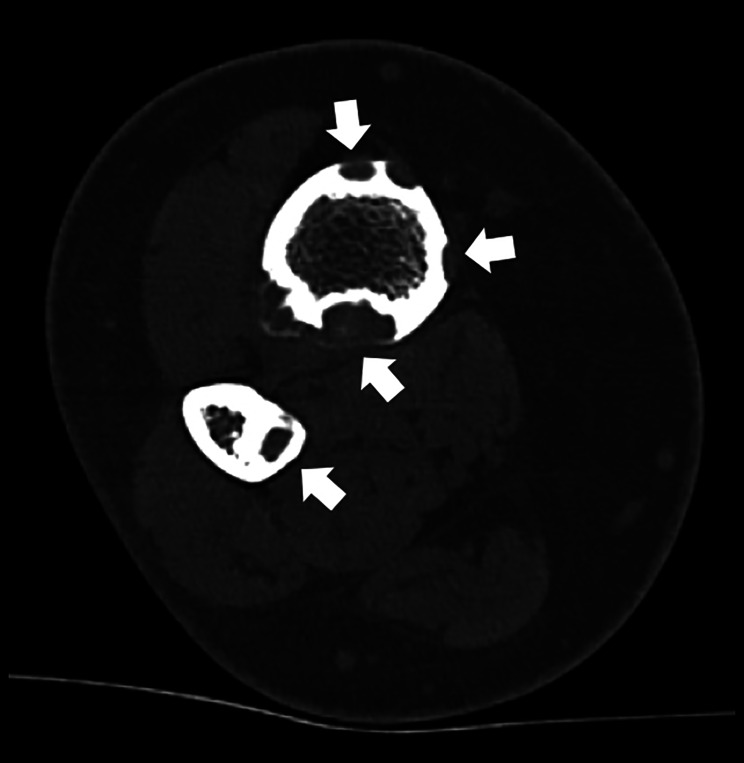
Axial CT image of the distal right leg showing well‑defined intracortical lucencies (arrows). Associated findings include cortical thinning and focal cortical defects, without evidence of periosteal reaction or extraosseous extension.
